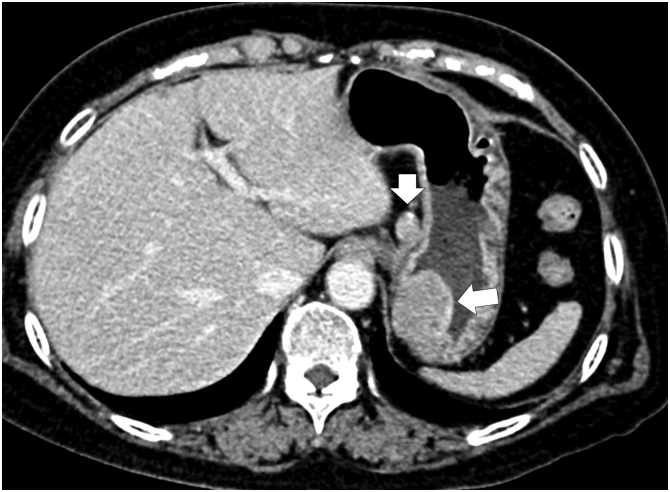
Abdominal computed tomography. A submucosal tumor 30 mm in diameter is connected to the gastric wall, and a 14 mm solid mass shows contrast effect in the early phase.
